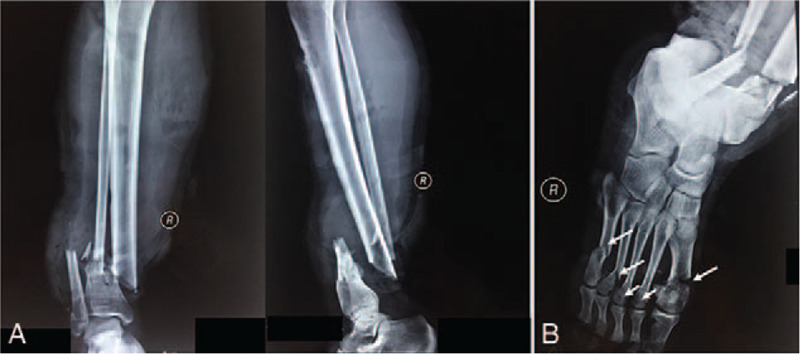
Digital radiograph of the patient's right lower extremity, demonstrating open tibial, fibular, and metatarsal fractures with an intact ankle complex, which conforms to the definition of a floating ankle. The anterior and lateral planes of the tibia and fibula demonstrated an AO 42b3 fracture with anterior dislocation (A). Digital radiograph of the right foot showing fracture of all metatarsals (B, arrows). The ankle mortise view remained intact (A and B).
